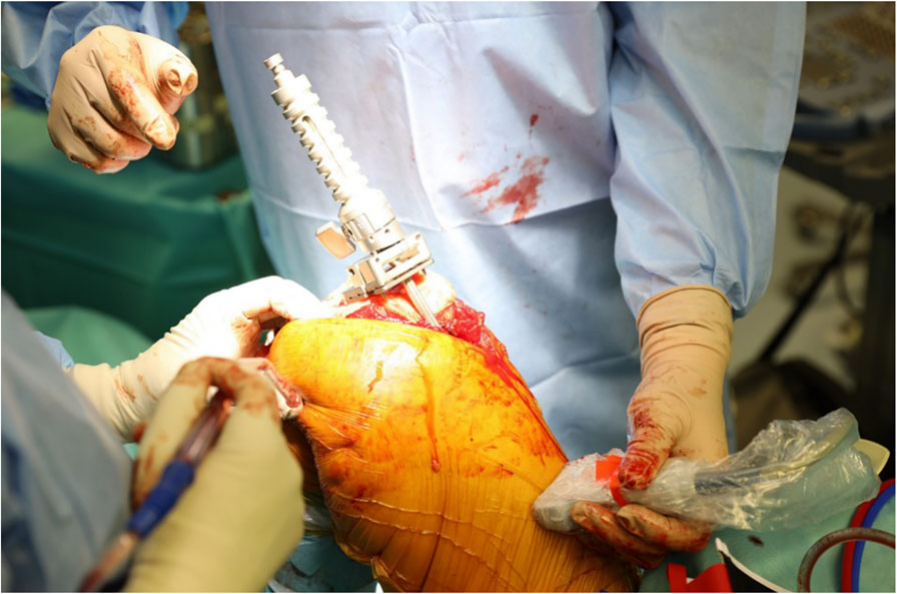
Ultrasound head placement to confirm extramedullary guide positioning
